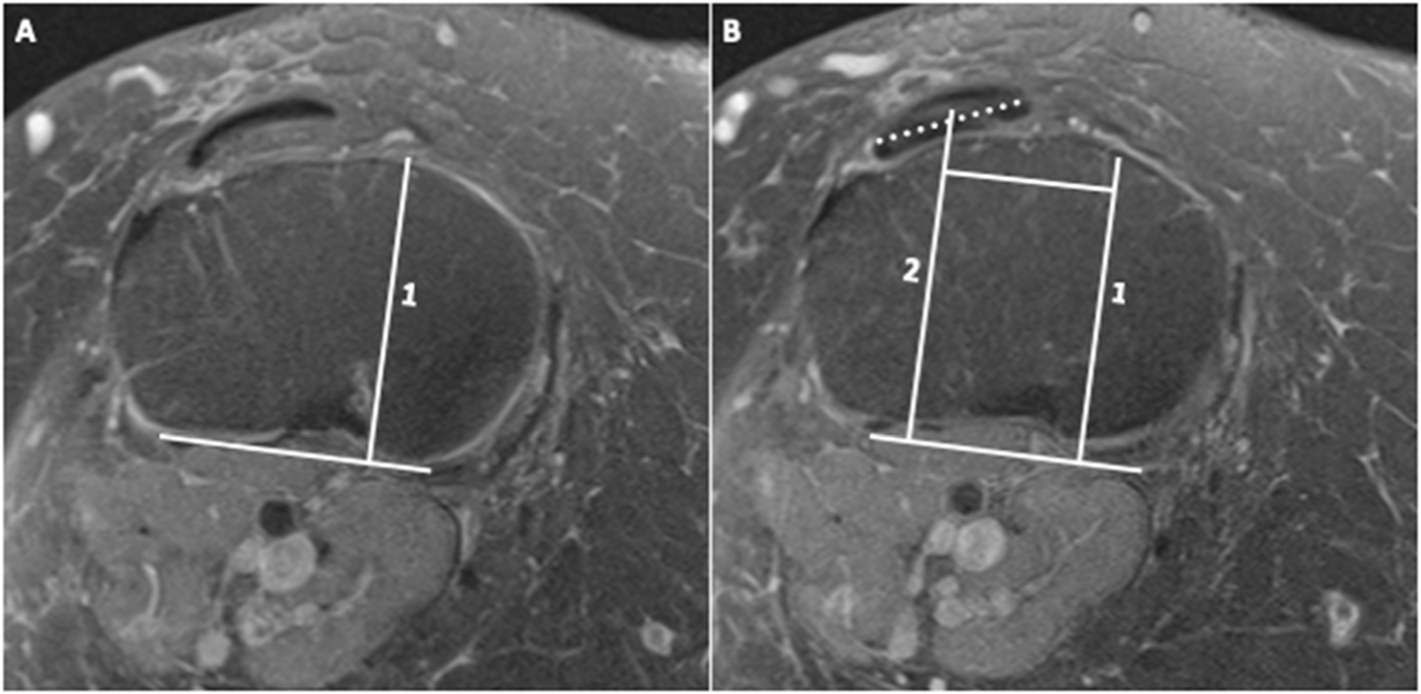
PD MR images of a 32-year-old female subject. a Line 1 was drawn through the medial border of the posterior cruciate ligament to form a 90° angle with the posterior tibial condylar line. b Line 2 was drawn in parallel to line 1 from the center of the patellar tendon (closest to the tibial tubercle) extending to the original posterior condylar line. The distance between lines 1 and 2 represents the PT-PCL
